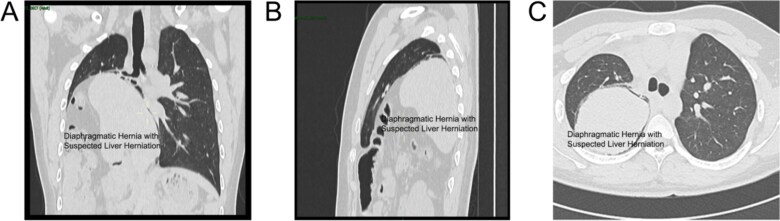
CT scan views of the thoracic hernia with suspected liver tissue involvement: (A) Axial view. (B) Frontal view. (C) Sagittal view.
